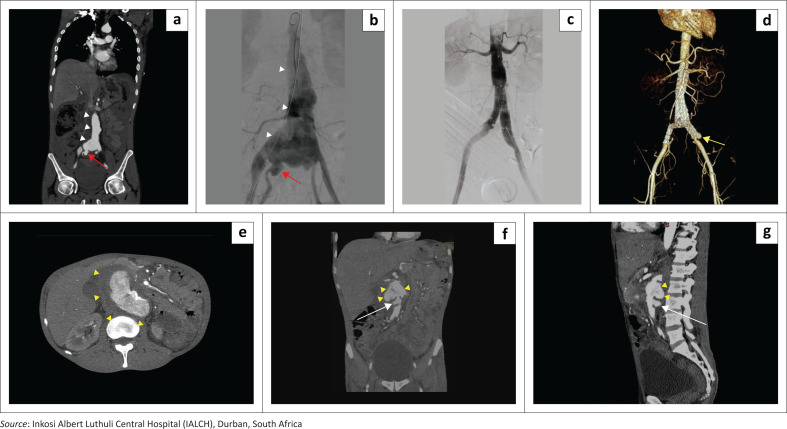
Multi-detector CT and angiographic images demonstrate variable aneurysm morphology without mural calcification in HIV-associated large-vessel vasculopathy. Panels (a–d) are from a 33-year-old man newly diagnosed with HIV who presented with acute abdominal pain and a pulsatile mass. (a) Contrast-enhanced coronal CT shows a large infrarenal fusiform abdominal aortic aneurysm (white arrowheads) with extension into the iliac arteries, and an associated saccular right internal iliac artery aneurysm (*red arrow*). (b) Digital subtraction angiography confirms the aneurysmal morphology. (c) Completion angiogram following bifurcated endovascular aneurysm repair with coiling of the aneurysmal sac demonstrates satisfactory exclusion. (d) One-year post-procedure CT angiographic 3D reconstruction shows interval development of a left common iliac artery aneurysm (yellow arrow) after defaulted follow-up. Panels (e–g) are from a different patient, a 42-year-old man, demonstrating multiple irregular, lobulated saccular aneurysms of the infrarenal abdominal aorta, with the largest (*yellow arrowheads*) revealing contained rupture. Owing to the extent of disease, surgical intervention was not performed.
